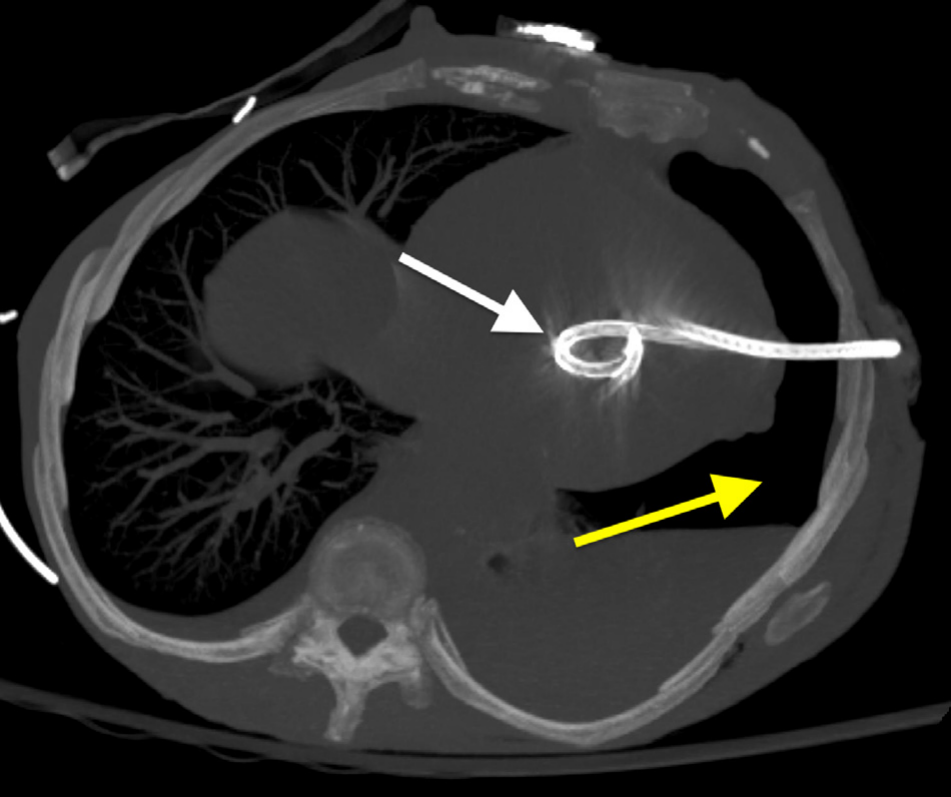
Axial MIP (Maximum Intensity Projection) CT image of the chest showing the location of the intracardiac chest tube (white arrow). CT imaging in Fig. 3, Fig. 4 played a vital role in quickly and accurately diagnosing the malpositioned chest tube and providing key information for the patient’s subsequent operation.
